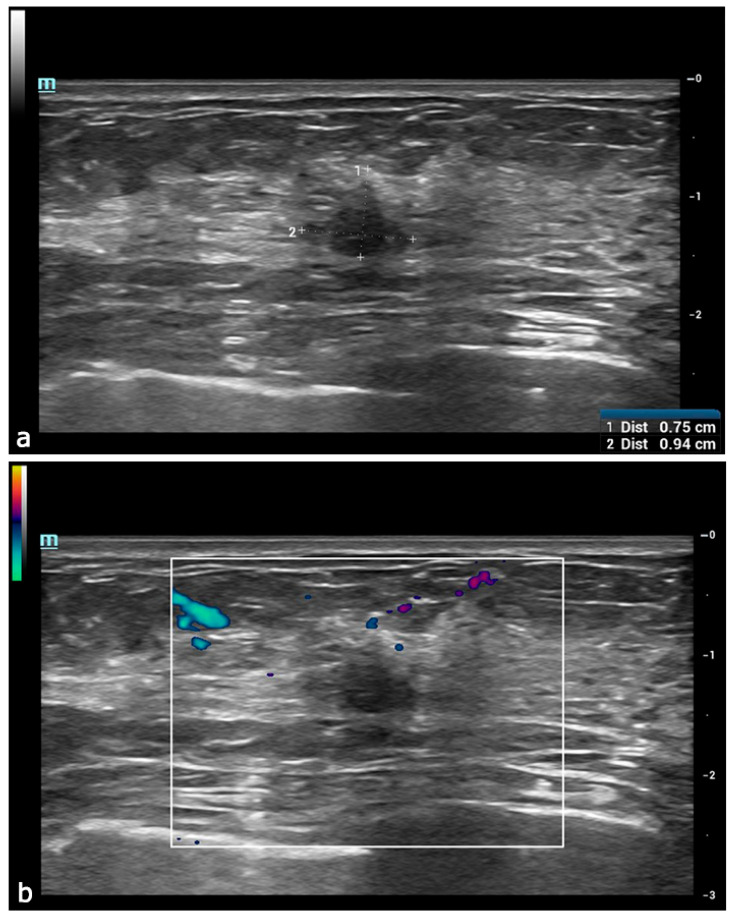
Subsequently, the patient underwent a multiparametric US examination, which included B-mode, Color Doppler, Strain Elastography (SE), Shear Wave Elastography (SWE), and contrast-enhanced ultrasound (CEUS). B-mode ultrasound of the UIQ of the left breast (a) revealed a hypoechoic irregular-shaped lesion with microlobulated margins and anti-parallel orientation, measuring approximately 9 × 7 mm. No intralesional vascular signals were found at the Color Doppler evaluation (b). No other suspicious lesions were detected, identifying the lesion as solitary. Given these findings, benign lesions were considered less likely in the differential diagnosis, with the main differential being between a primary breast lesion and a metastatic tumor from a NET, despite the latter being extremely rare. The lesion was classified as BI-RADS category 4C, indicating a moderate suspicion of malignancy and warranting a US-guided core biopsy.
